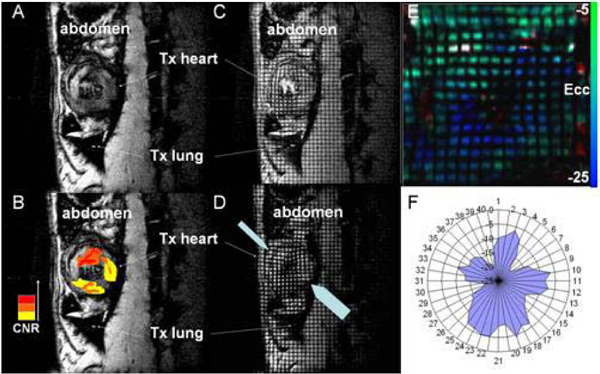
(A) T2*-weighted MRI for an allograft after USPIO administration. (B) Pseudo-coloring of different contrast-to-noise ratios (CNR) for easier visualization. (C, D) Tagged MRI of the same allograft heart at ED (C) and ES (D). The block arrows point to regions with compromised contractile functions. (E) Colored Ecc strain map of the allograft. (F) Ecc stran values of 48 probe-points throughout LV.
